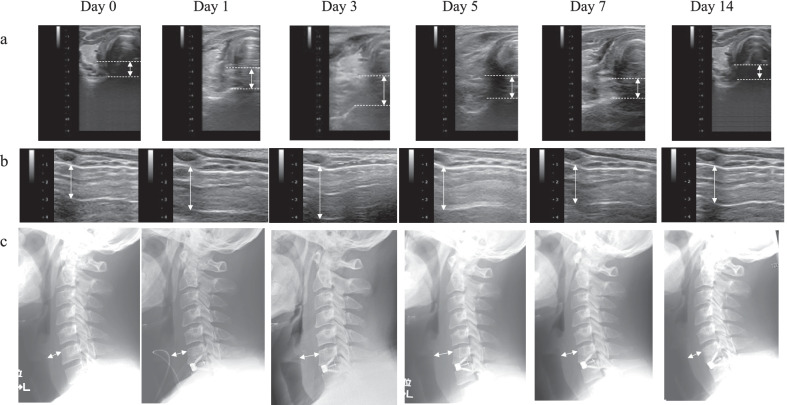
Postoperative course of the prevertebral soft tissue (PST) and upper airway in the representative case. a: Postoperative changes in the thickness of the PST measured via ultrasonography. b: Postoperative changes in the upper airway measured via ultrasonography. c: Postoperative changes in the thickness of the PST measured via lateral radiography of the cervical spine
